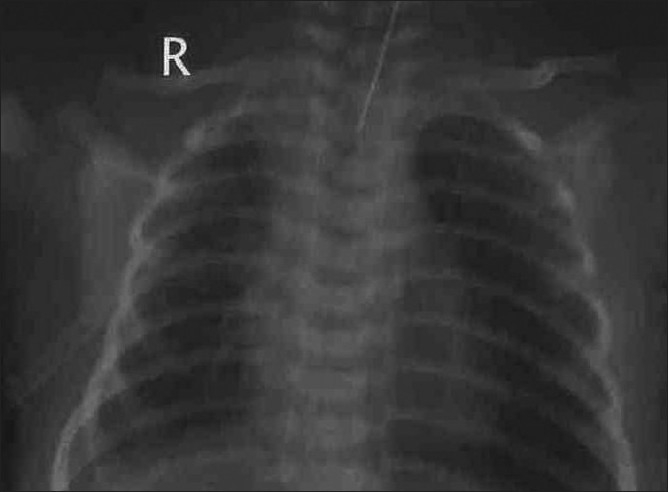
Follow-up chest radiograph showing full expansion of lung fields immediately after chest tube drain
